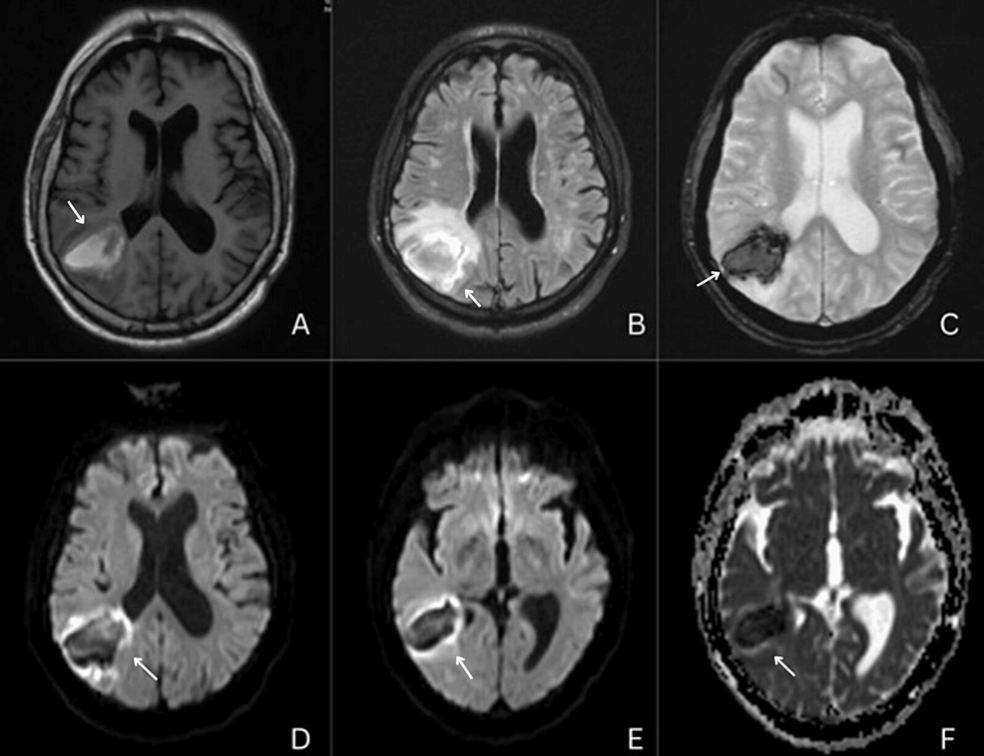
Different MRI sequences showing the right intraparenchymal hemorrhage A: T1; B: Fluid Attenuation Inversion Recovery (FLAIR); C: Gradient Echo Sequence (GRE); D, E: Diffusion Weighted Imaging (DWI); F: Apparent Diffusion Coefficient (ADC). (White arrows show the right intraparenchymal hemorrhage in the above images).
